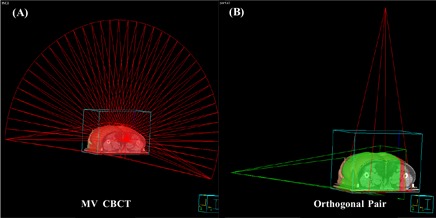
(A) The room's eye view of a megavoltage cone‐beam computed tomography image, created by delivering an arc beam from 270 degrees to 110 degrees, clockwise direction. (B) The room's eye view of orthogonal portal images with 0‐degree (anterior–posterior) and 90‐degree or 270‐degree (lateral) acquisition as an orthogonal pair.
